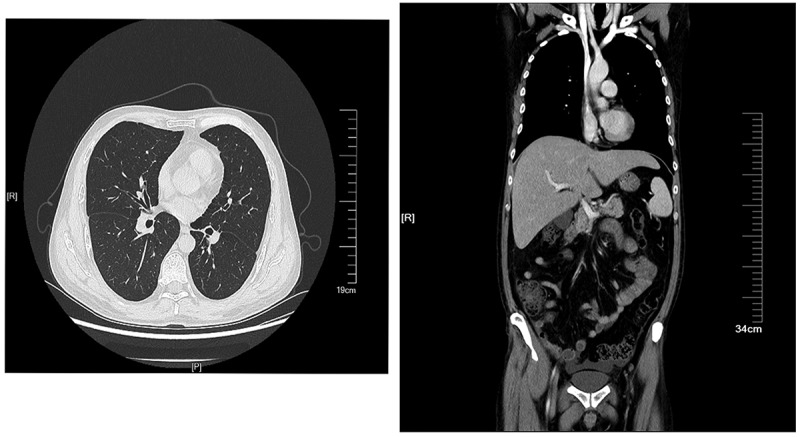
A CT scan of chest and abdomen on the day of admission. A mild inflammation was seen in the lower lobes of bilateral lungs, and the liver was slightly enlarged.
